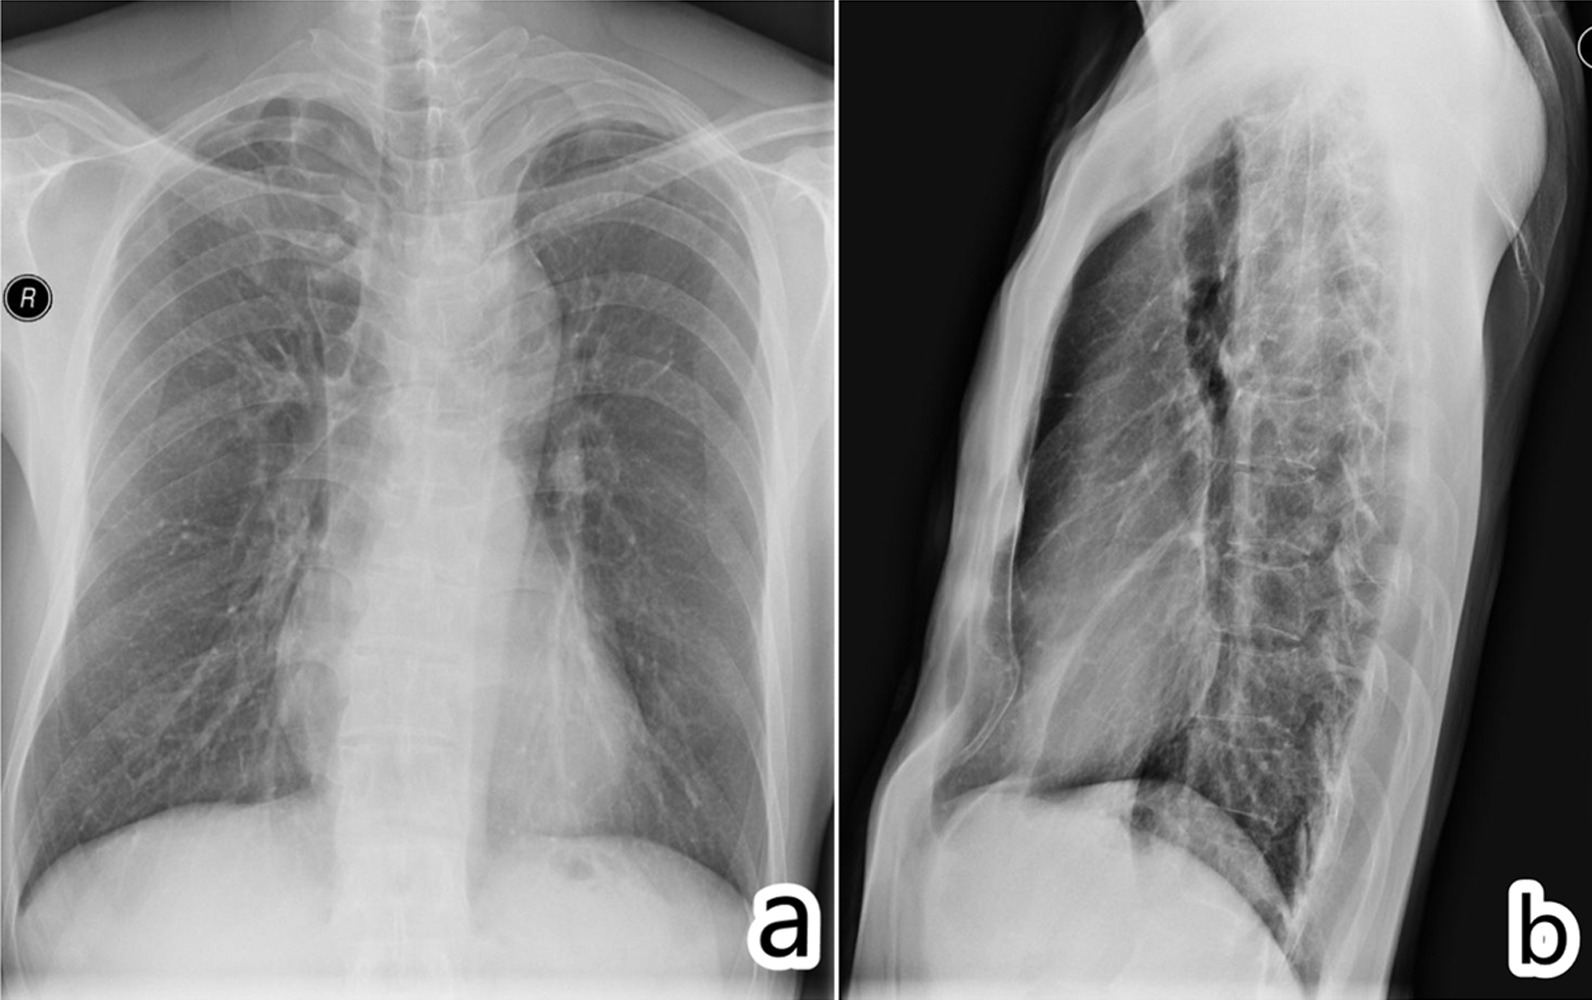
Chest radiographs. a Bilateral bullae, scoliosis; b Pectus excavatum
